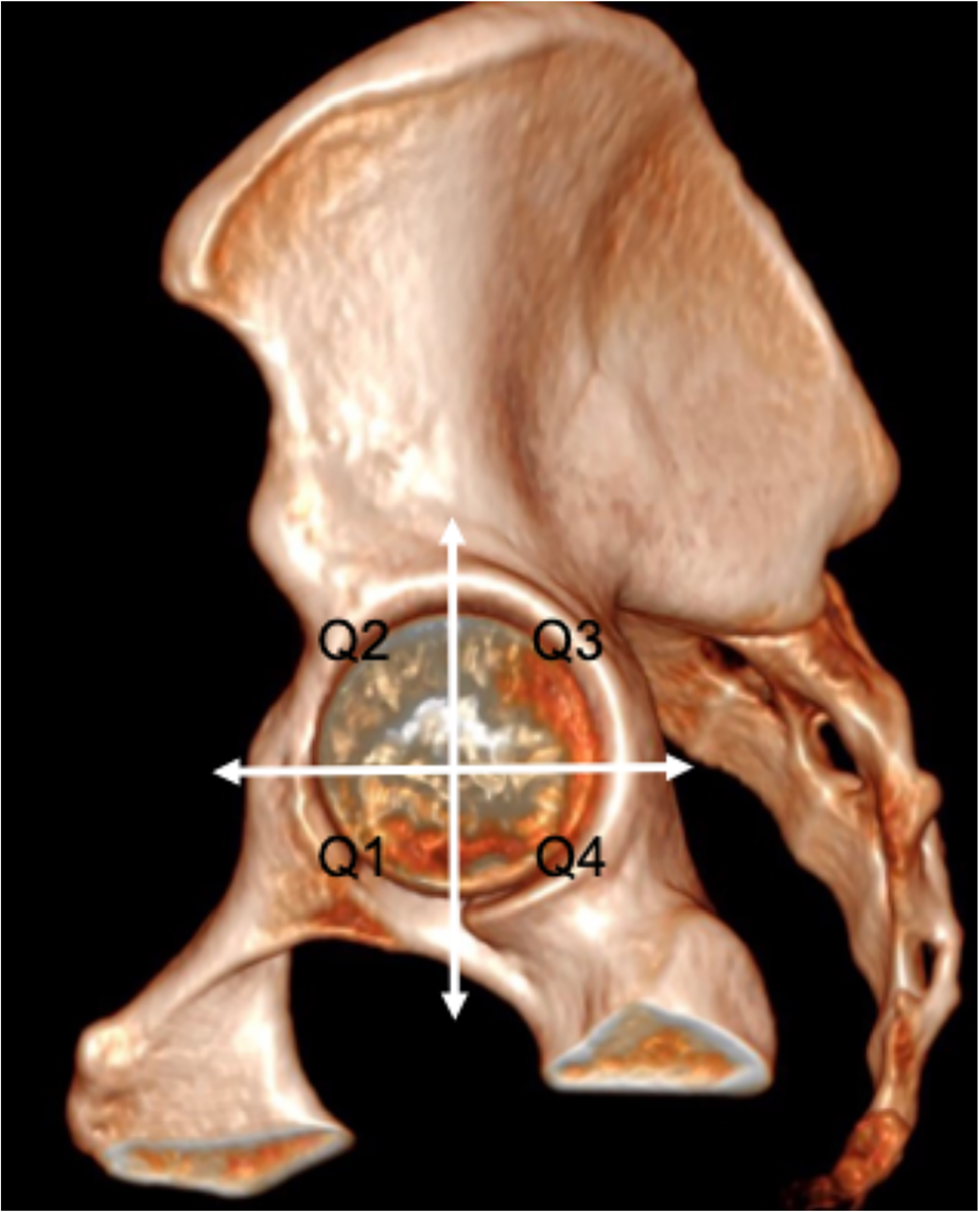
Volume rendering reconstruction of CT of left hip joint showing the location of the quadrants around the acetabular rim. (Q1) Anteroinferior quadrant, (Q2) anterosuperior quadrant, (Q3) posterosuperior quadrant, and (Q4) posteroinferior quadrant
